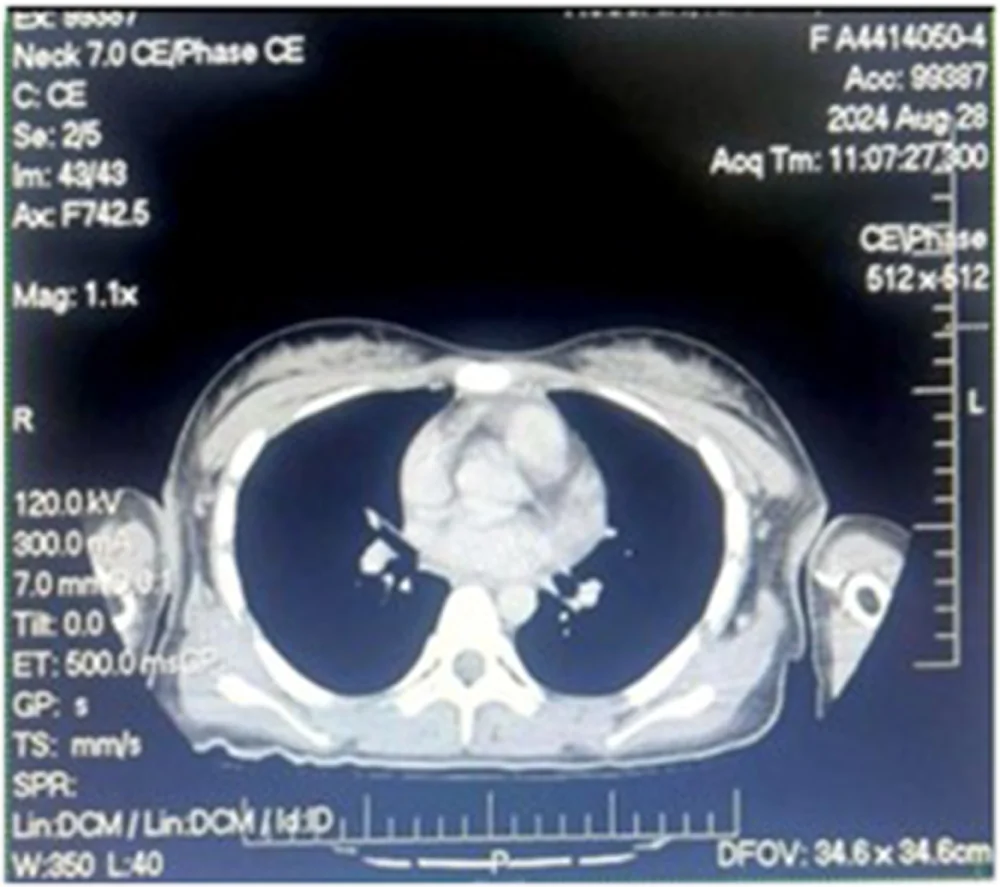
Axial contrast-enhanced CT image of the chest demonstrates both lungs as well-aerated, low-density areas on either side, with the heart and mediastinal structures centrally located and opacified blood vessels due to contrast administration. The cardiac silhouette and major vessels are clearly visualized, the vertebral body is seen posteriorly, and the ribs and chest wall are intact. No obvious large mass, gross consolidation, or significant pleural fluid is apparent.
